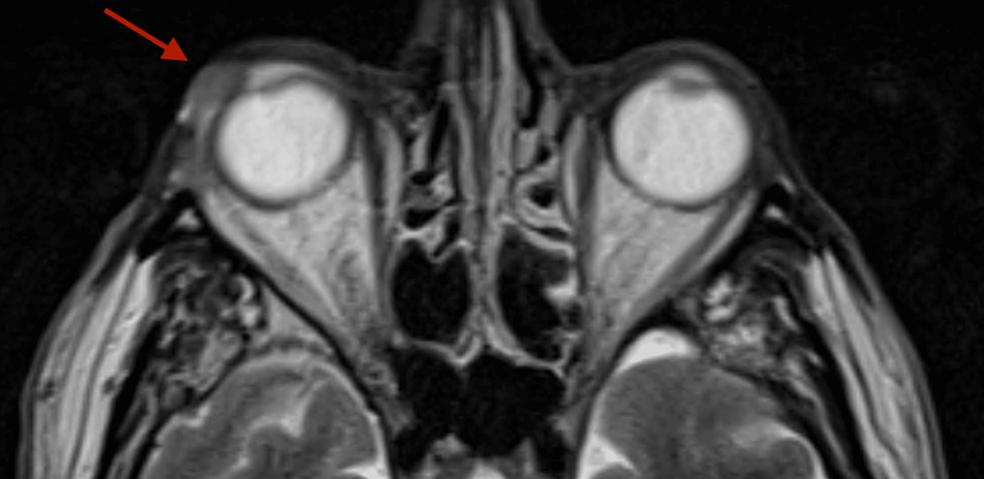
MRI of the brain and orbits showing a lesion in the anterolateral quadrant of the right eye (red arrow).
